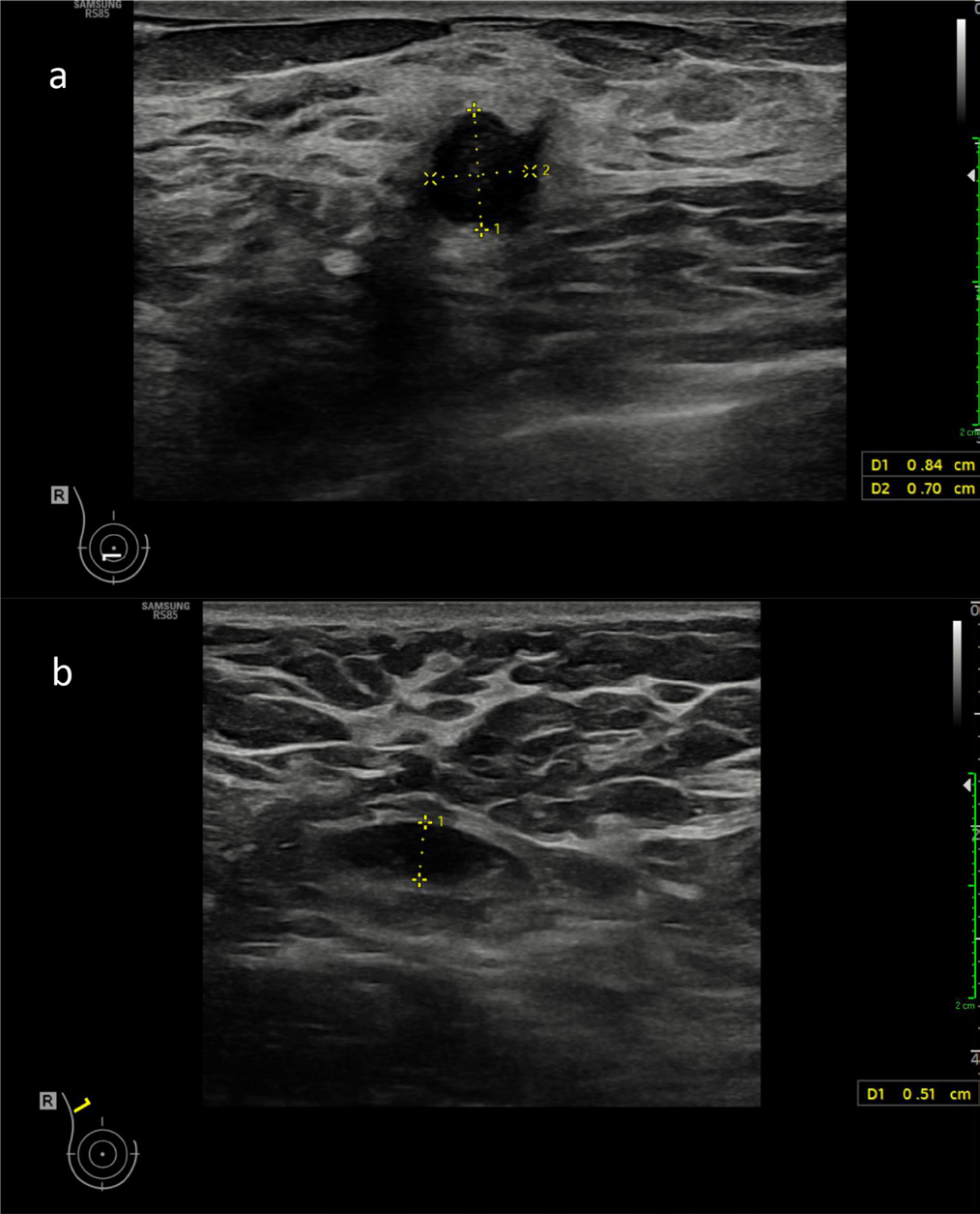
(a) Ultrasound image demonstrating an 8 × 7 mm necrotic
